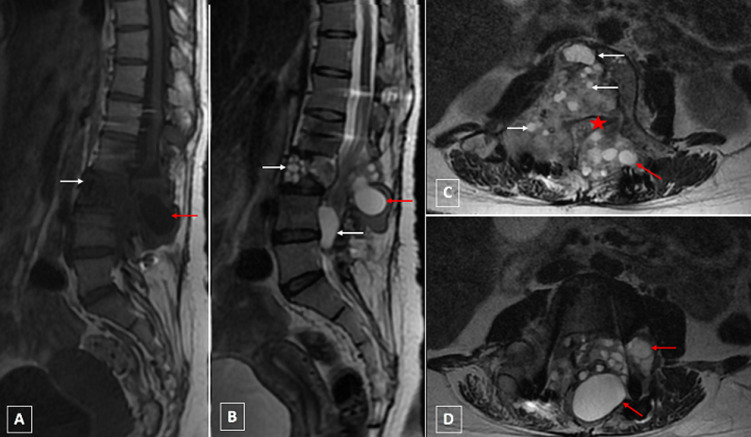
lumbar MRI in sagittal (A,B) and axial sections (C,D) respectively: A (T1WI) and B,C,D (T2WI) showing at L3 level expansible heterogenous lesion with multiple cysts presenting low signal on SE T1 WI (A) and high signal on SE T2 WI (B,C,D), with extension into the spinal canal and compression of the cauda equina (star) and into the peri vertebral soft tissue (red arrow): dumbbell formation according to Braithwaite and Lees classification
